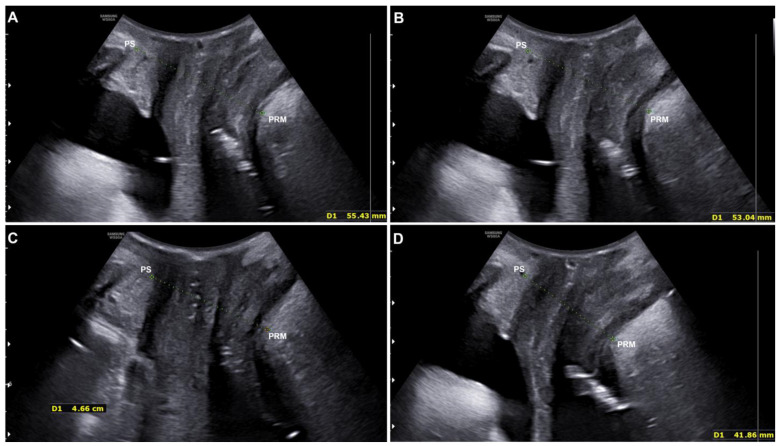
Ultrasound evaluation of a patient at rest before (A) and after (B) the treatment with electromagnetic stimulation. The ultrasound evaluation performed on the same patient under stress/contraction condition before (C) and after (D) the treatment with electromagnetic stimulation. The green lines represent the distance between the PS (pubic symphysis) and the PRM (puborectalis muscle).
